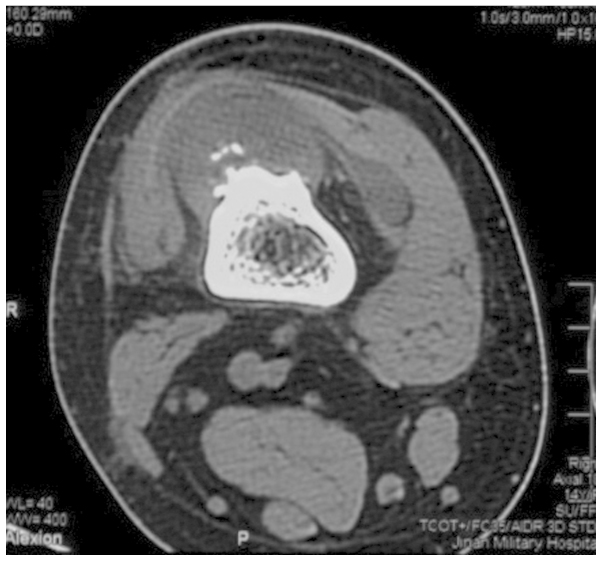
Computed tomography revealed a solid tumor of 5×4 cm in diameter and associated with the right distal femur bone, with abundant specks of calcification.
